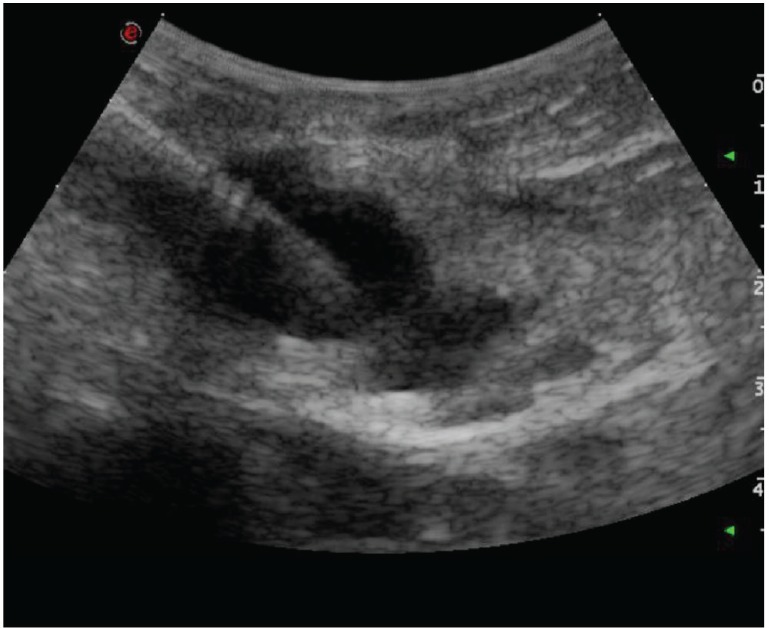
Ultrasound-guided biopsy of an omental nodule, whose diagnosis was suspicious for carcinoma with the unknown primary site.
